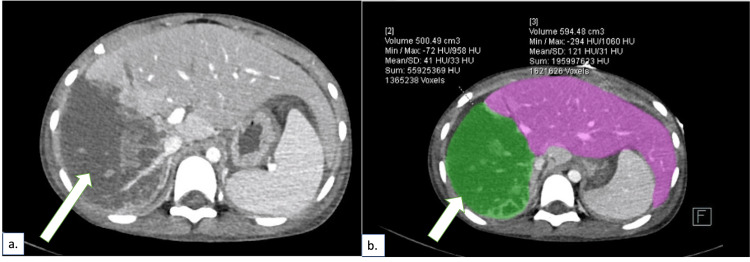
Contrast-enhanced computed tomography on post-injury day 5 showing a nonenhancing right lobe (arrow) similar to that at presentation (Figure 1): (a) axial section; (b) volumetric analysis of the involved area of the liver (green).
